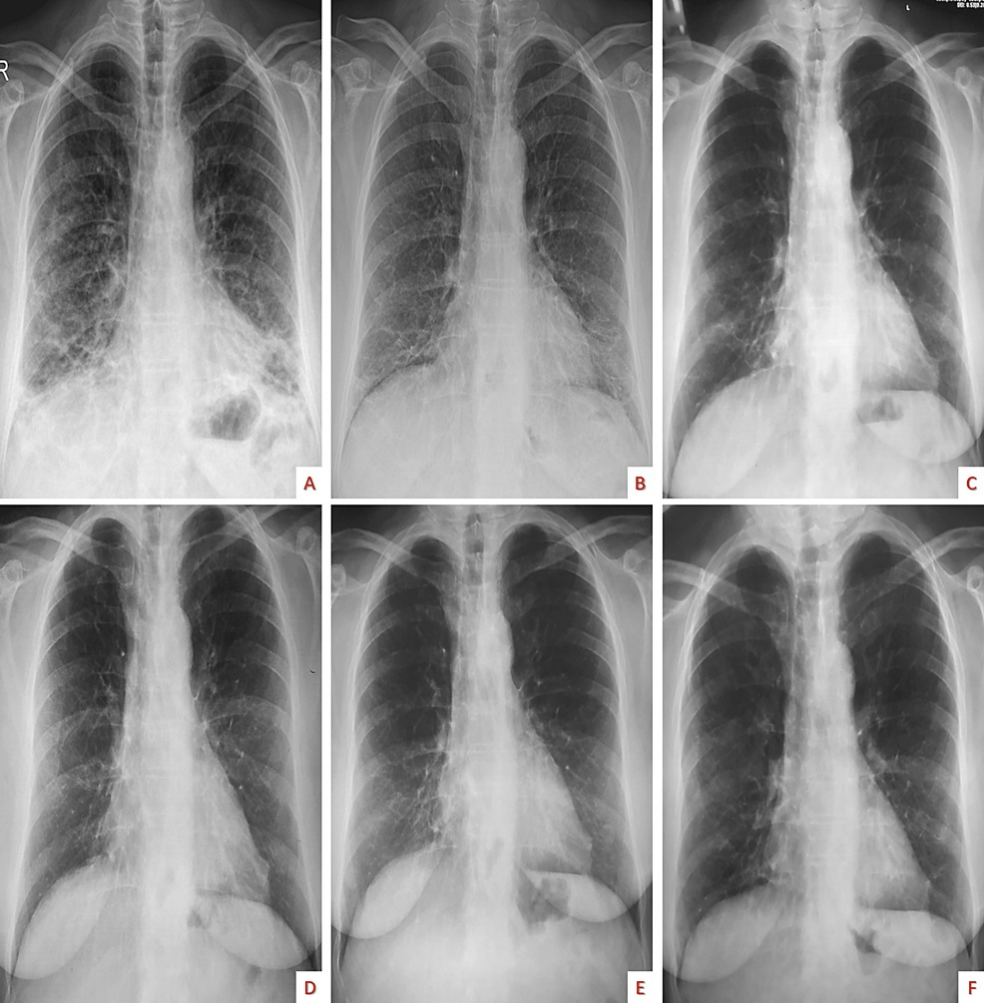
Serial chest X-rays showing a radiological improvement Steroid naive (A), after eight days of therapy (B), after two months of therapy (C), after three months of therapy (D), after six months of therapy when corticosteroids stopped (E), after seven months from admission and one-month corticosteroid free (F).
